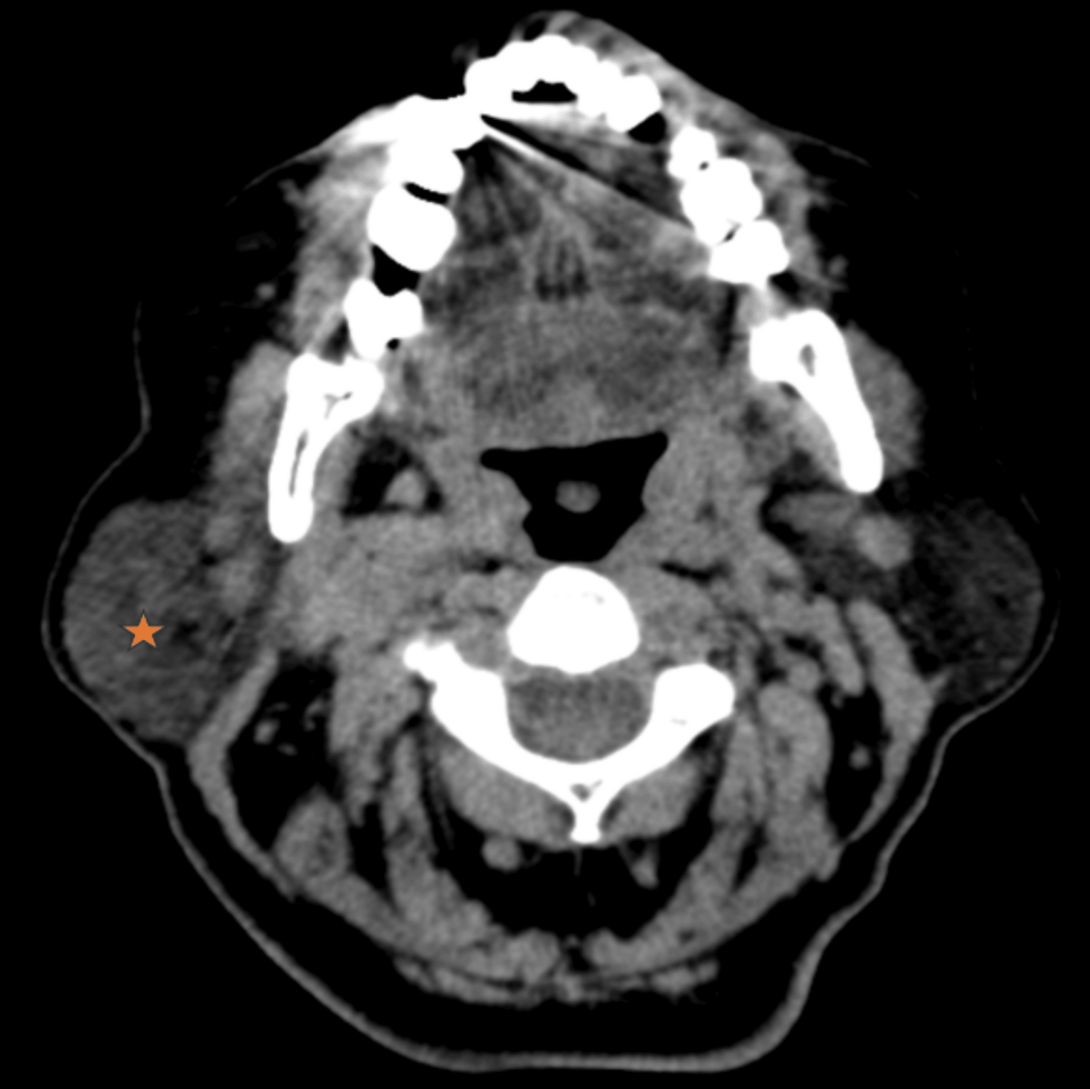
Contrast-enhanced CT of the neck showing right lobulated parotid mass (star). CT: computed tomography
